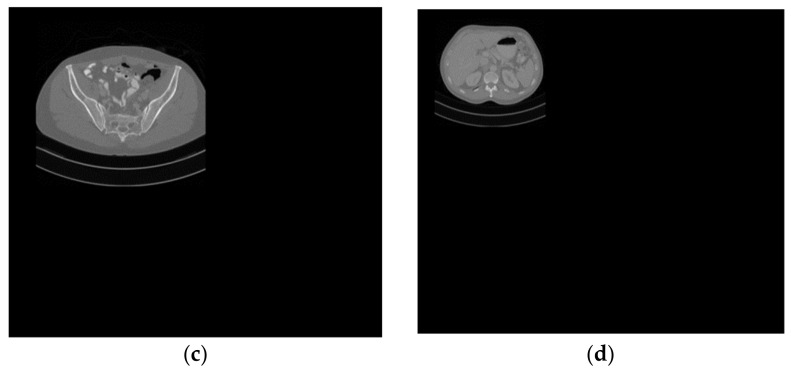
Expanded medical CT images. (a) Expanded “001” image, (b) Expanded “002” image, (c) Expanded “003” image, (d) Expanded “004” image.
